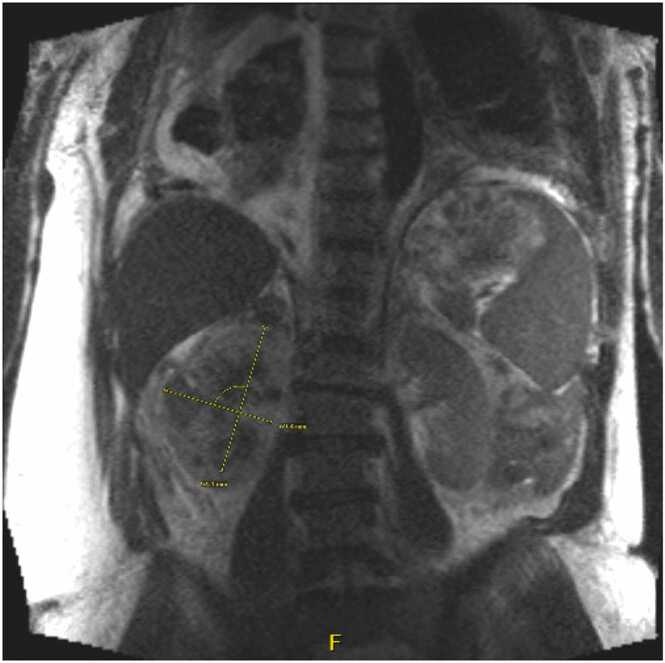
MRI abdomen coronal demonstrating a 9 cm soft tissue mass encasing the kidney with extension along the renal vasculature. Abbreviations: MRI, magnetic resonance imaging.
